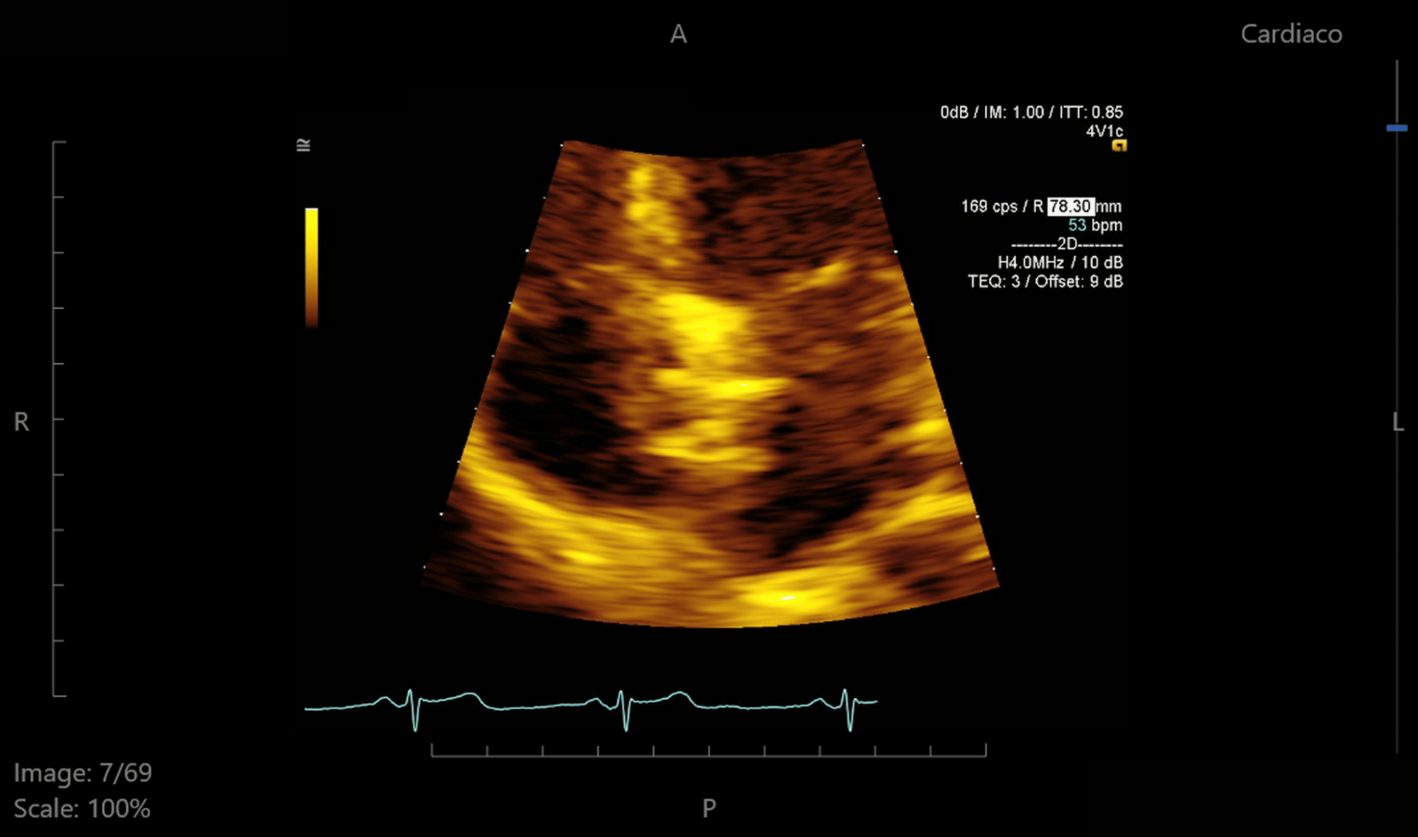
Zoomed apical four-chamber view highlighting the Gore Cardioform device at the interatrial septum, confirming its position and stability.
